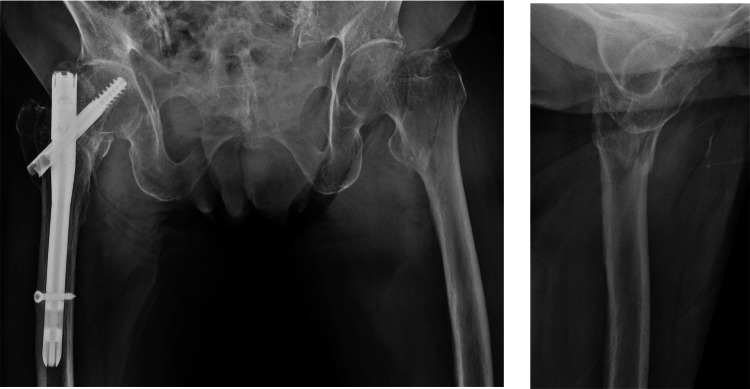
Pre-operative X-ray images of the hip. A fracture line was visible in the intertrochanteric region of the left proximal femur, with minimal displacement.
